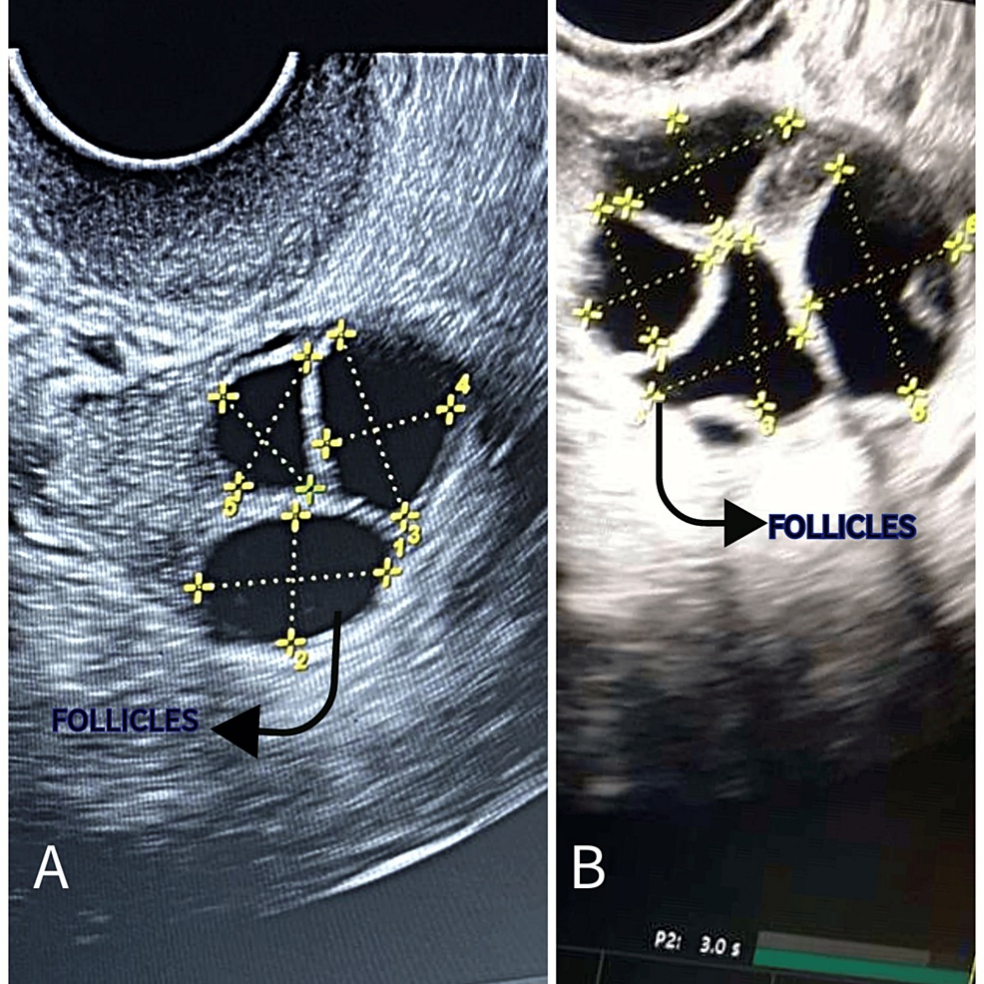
Ultrasonographic image of the left ovary showing follicles. (A) Before PRP therapy. (B) After platelet-rich plasma therapy. The original picture was captured by the author of this article, and informed consent was obtained for publication from the patient. PRP, platelet-rich plasma
